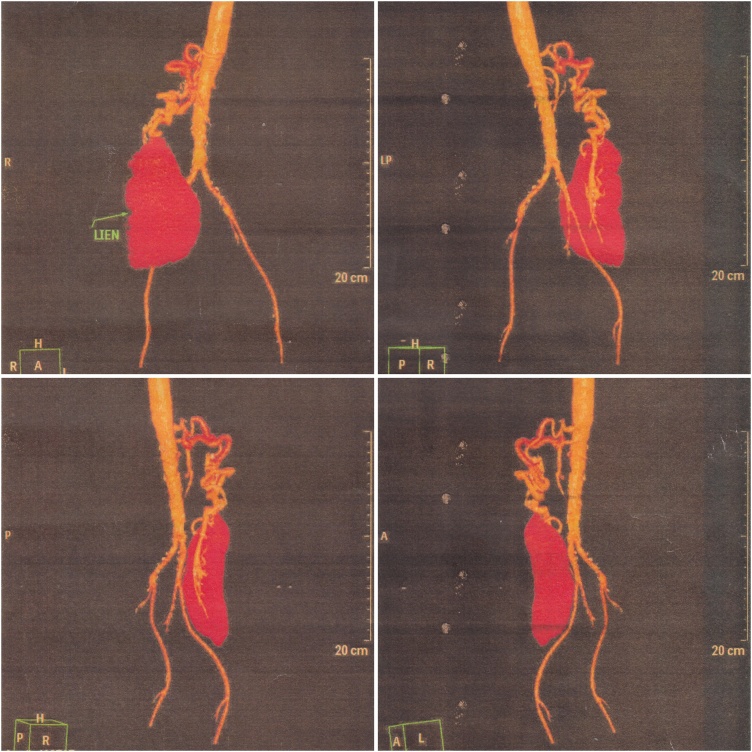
Volume-rendered technique CT abdominal angiography showing an anticlockwise torsion of the elongated splenic vascular pedicle in a whirlpool disposition.
